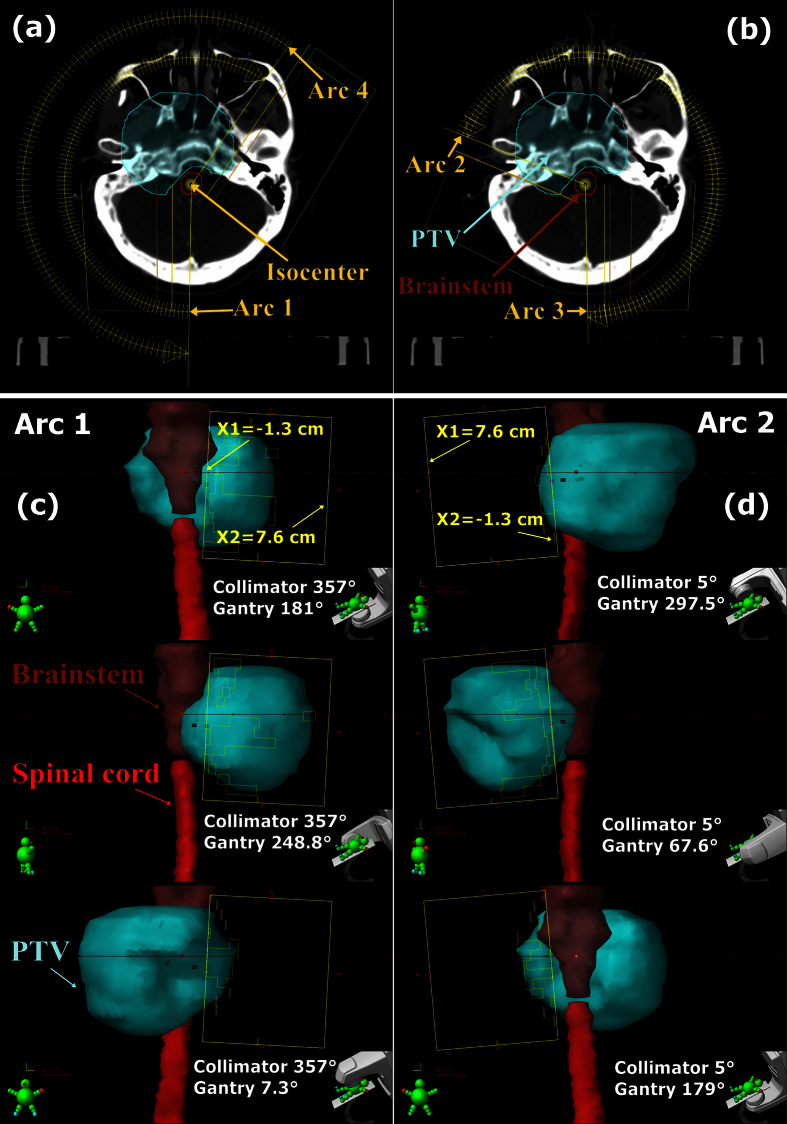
Geometry of the isocentrically shielded RapidArc (IS-RA) technique for a locally recurrent nasopharyngeal cancer (lrNPC) case in Stage rT4N0M0, with the volume of planning target volume (Vol_PTV) of 180 cm^3^, the angle extended by the PTV with respect to the axis of brainstem/spinal cord (Ang_BSSC) of 186° and the minimum distance between PTV and BS/SC (Dist_Min) of 0 mm. (a,b) Isocenter location and four partial arcs within two gantry rotations on an axial CT image; (c,d) the beam’s eye views (BEVs) in two partial arcs within one gantry rotation.
